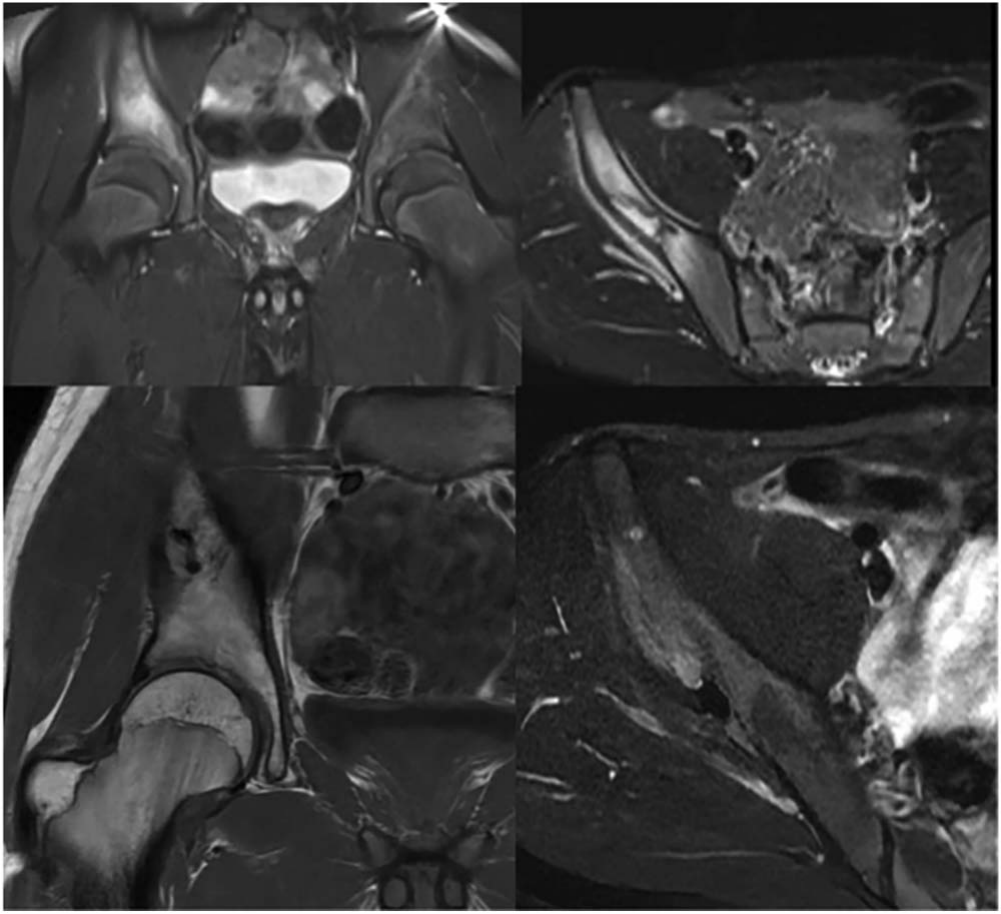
Preoperative and postoperative MRI images of a patient diagnosed with LCH in the iliac bone.
